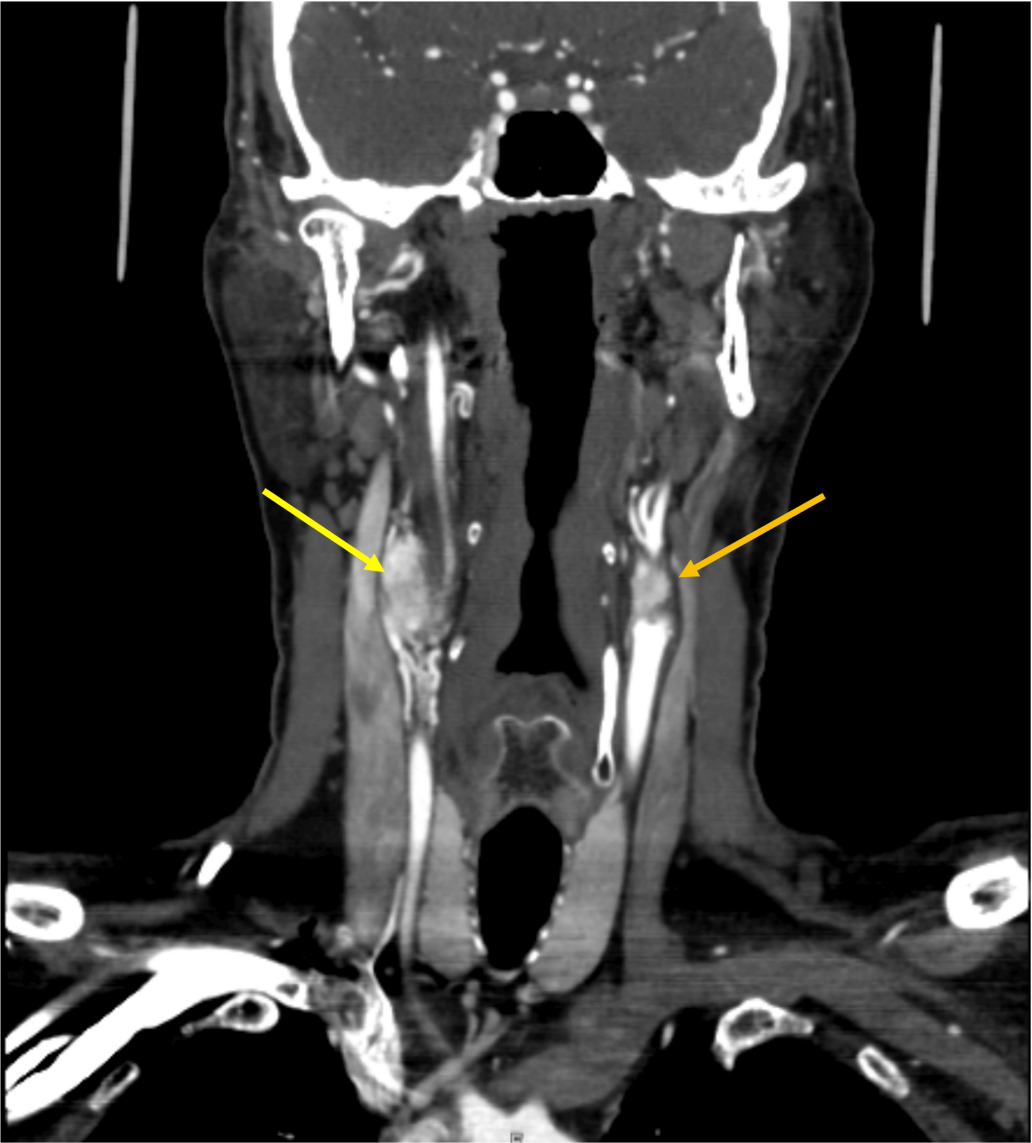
CT angiogram of the neck: Coronal section depicting the bilateral carotid body tumors encasing the internal and external carotid arteries on the right (yellow arrow) and abutting the arteries on the left (orange arrow).
